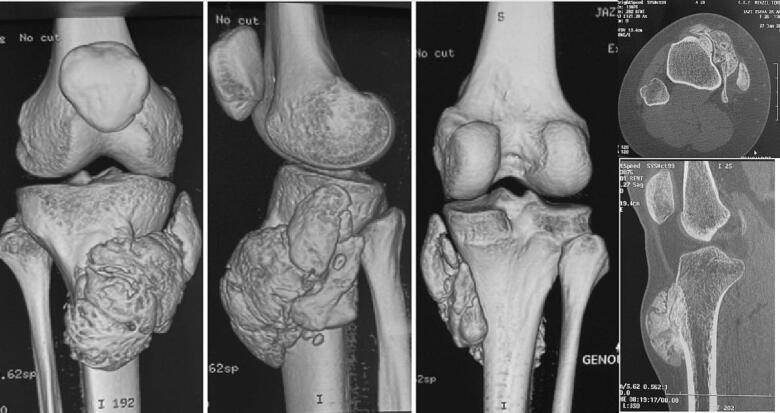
CT scans showing a tumor involving the cortical region without medullary extension.
